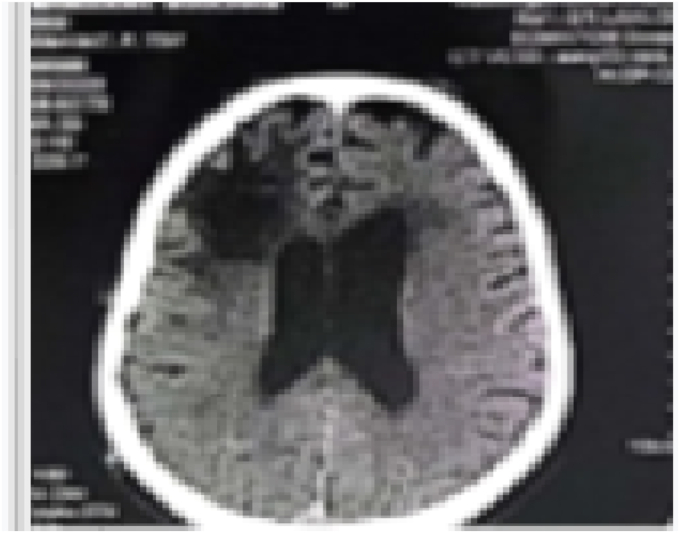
CT SCAN control 2 days after surgery no signs of tumor.
